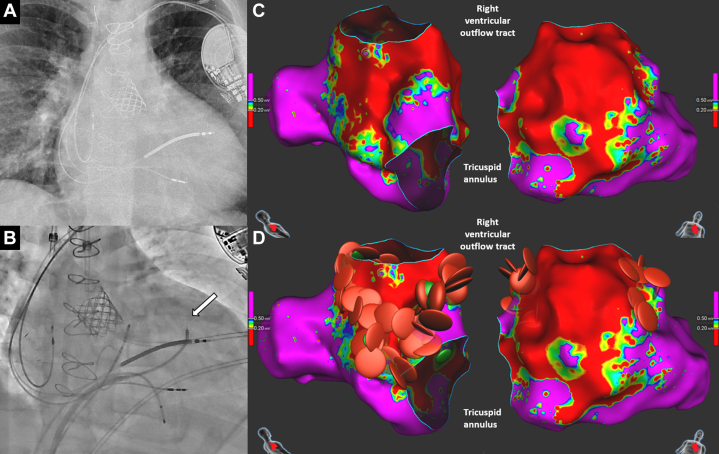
Findings on Chest X-Ray and Ablation Procedure in Case 3 (A) Anteroposterior chest x-ray showing cardiac resynchronization device and valve prosthesis in the right ventricular outflow tract. (B) Fluoroscopy image (right anterior oblique view) showing the position of the Sphere-9 catheter (arrow) against the high right ventricular septum. (C) Septal and lateral views showing scar areas. (D) Radiofrequency ablation lesions connecting the tricuspid annulus to the scar on the septal and lateral aspects.
